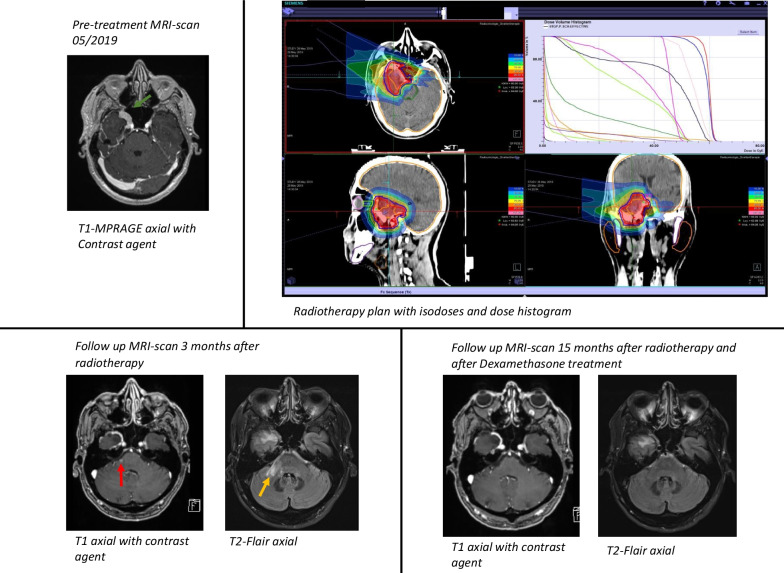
Case example. Images of patient A with a SFT of the sphenoid wing on the right side (green arrow). This patient underwent a biopsy and started proton radiotherapy 2 months after initial diagnosis. Response was excellent showing a CR and no recurrence during his 17 months of follow- up. Nevertheless, during follow-up, the patient suffered from RICE in the right pontine region (T1 contract enhanced (red arrow) with corresponding T2 FLAIR edema (yellow arrow), which resolved after dexamethasone therapy)
